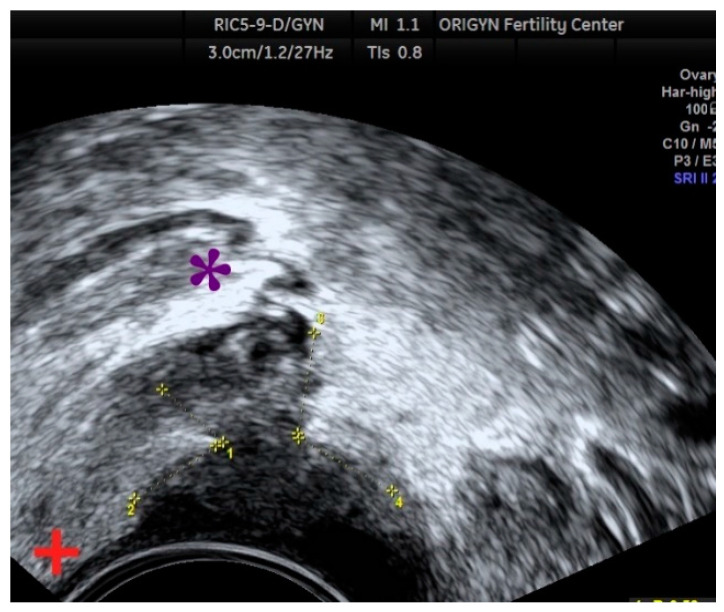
Ultrasound image showing a big nodule infiltrating the posterior vaginal fornix and the anterior wall of the rectum. (+) the vaginal wall, (*) the bowel. Diablo-like nodule.
